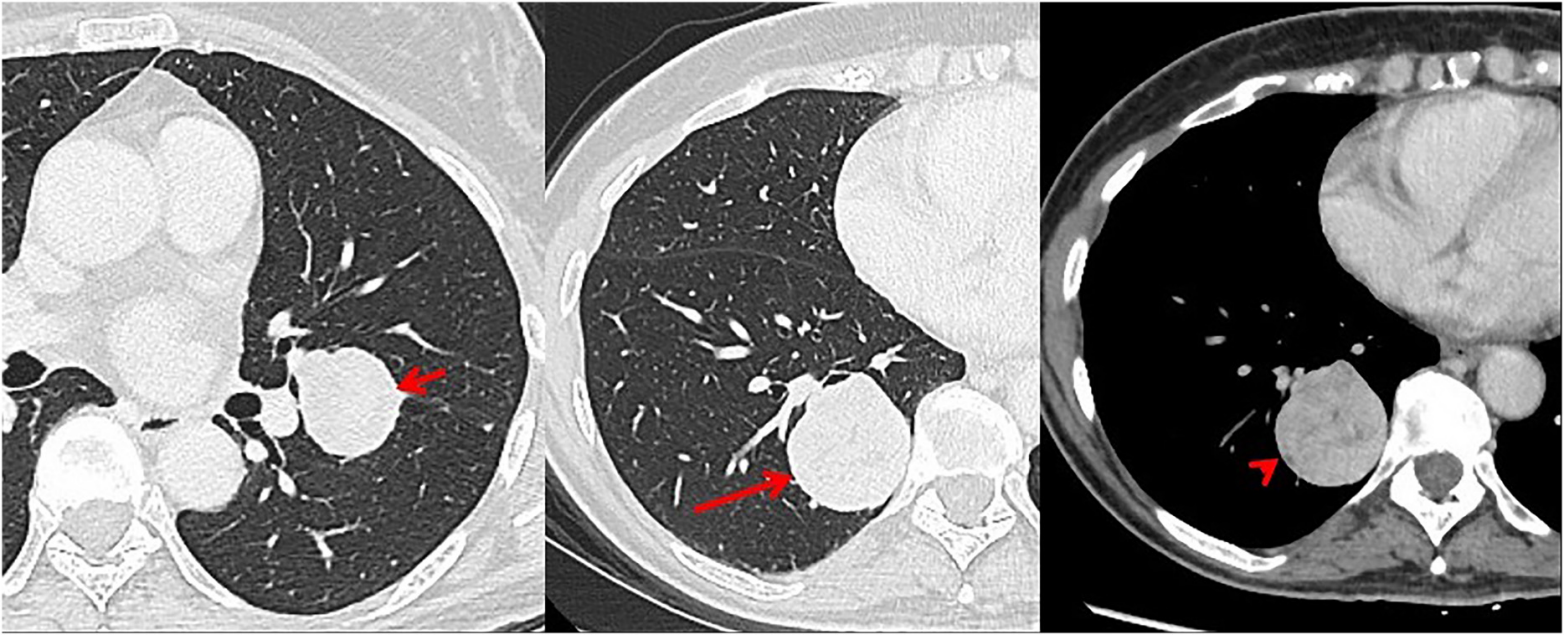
Computed tomography (CT) images in axial plane in lung window (A and B) show well
